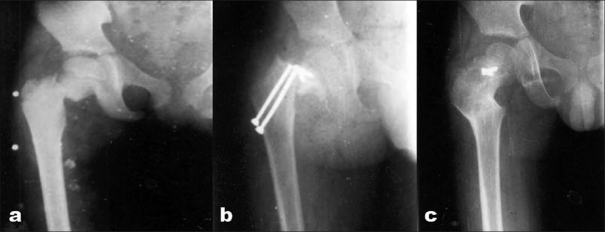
(a) Preoperative radiograph of right hip joint (anteroposterior view) of a 13-year-old boy showing a displaced femoral neck fracture who presented after 5 days. (b) X-ray hip joint (anteroposterior view) of the same patient showing breakage of implant at 18 weeks. (c) A radiograph hip joint (anteroposterior view) of showing union after implant (distal part) removal and valgus osteotomy
